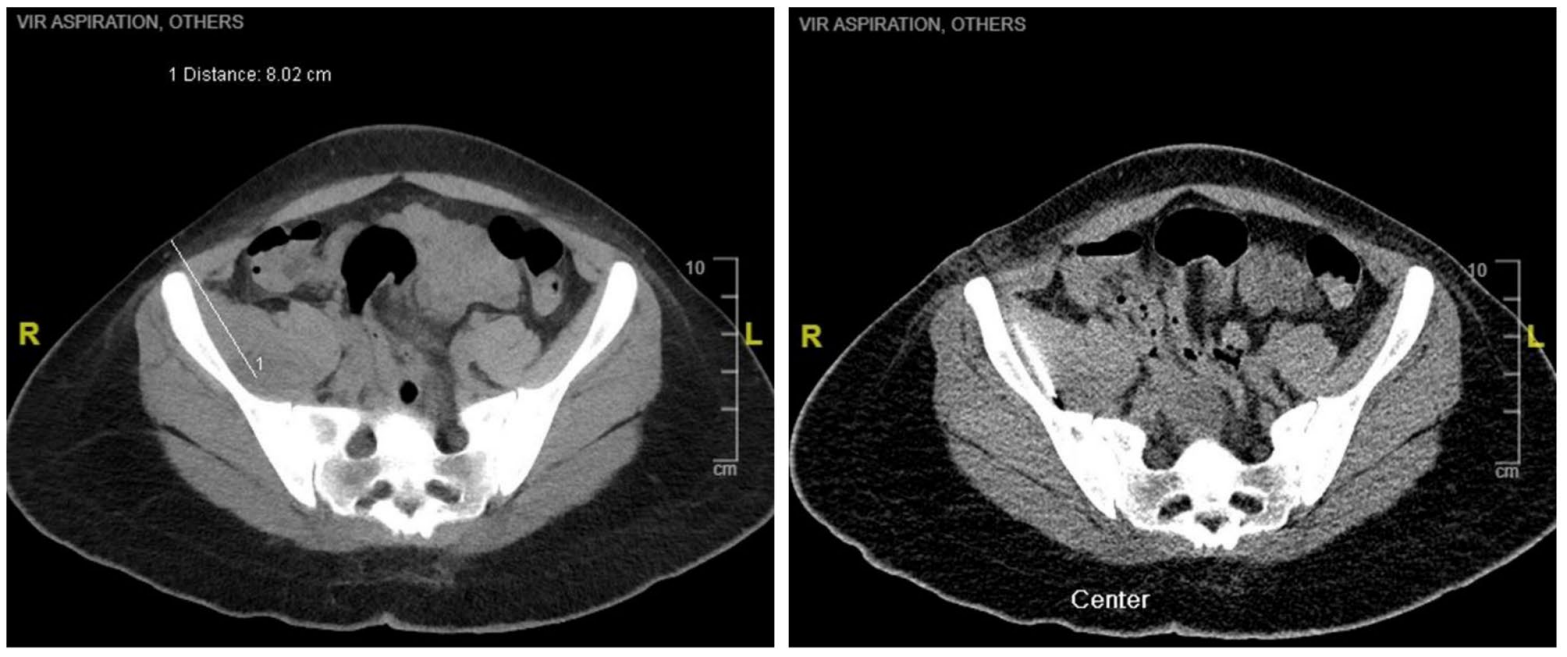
Axial view of computed tomography guided aspiration of the right iliacus muscle abscess located approximately 8.02 cm from the skin
